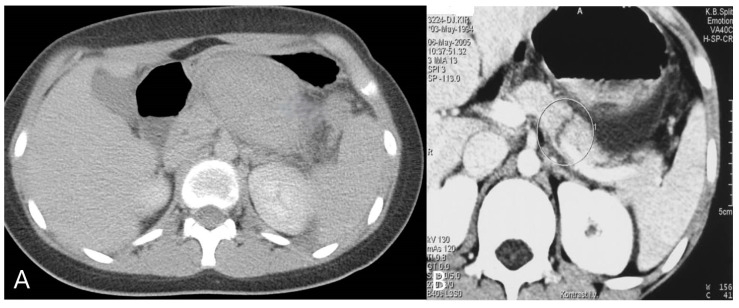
Pancreatic injury: (A) Post-contrast CT sections through the abdomen in the region of the omental bursa show a fluid collection corresponding to a hematoma of the omental bursa. (B) CT representation of pancreatic rupture.
